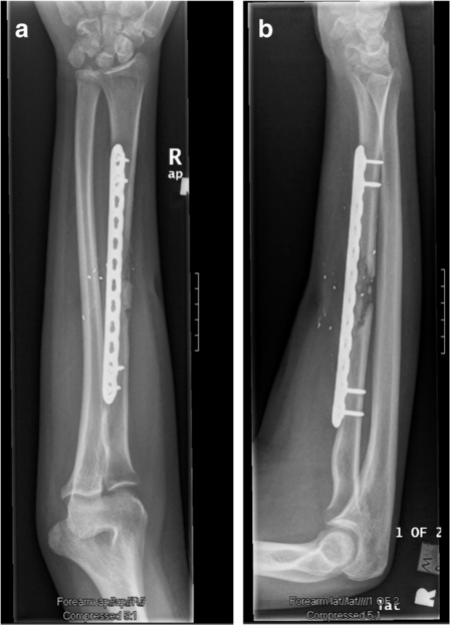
AP (a) and lateral (b) radiographs of the right forearm 2 months post-op.
